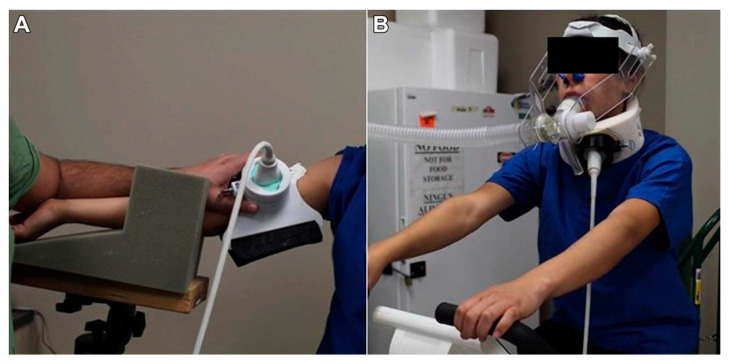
Blood flow pattern measurements using ultrasound imaging on the brachial (A) and carotid (B) arteries. Adapted from Gurovich et al., 2021 [10].
